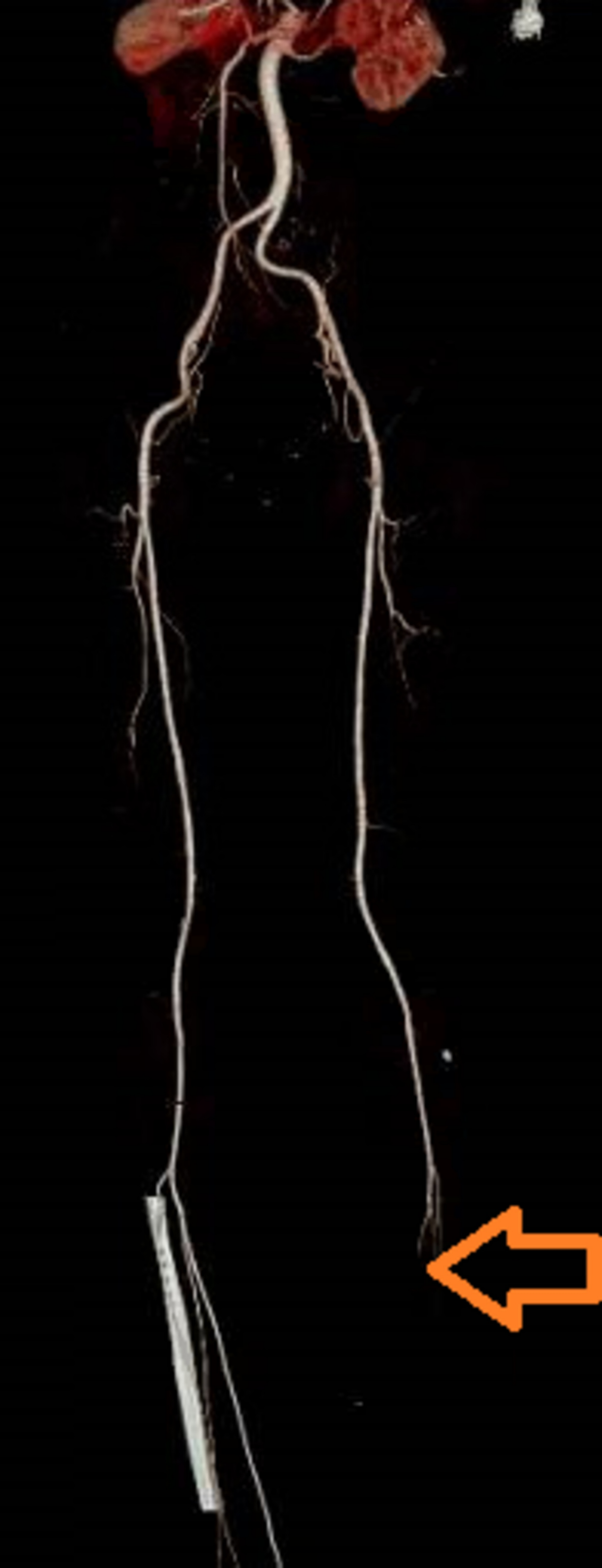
CT angiogram of the lower extremities The arrowhead showing reduced blood flow with blockage in the arterial vessels of the left lower extremity.
